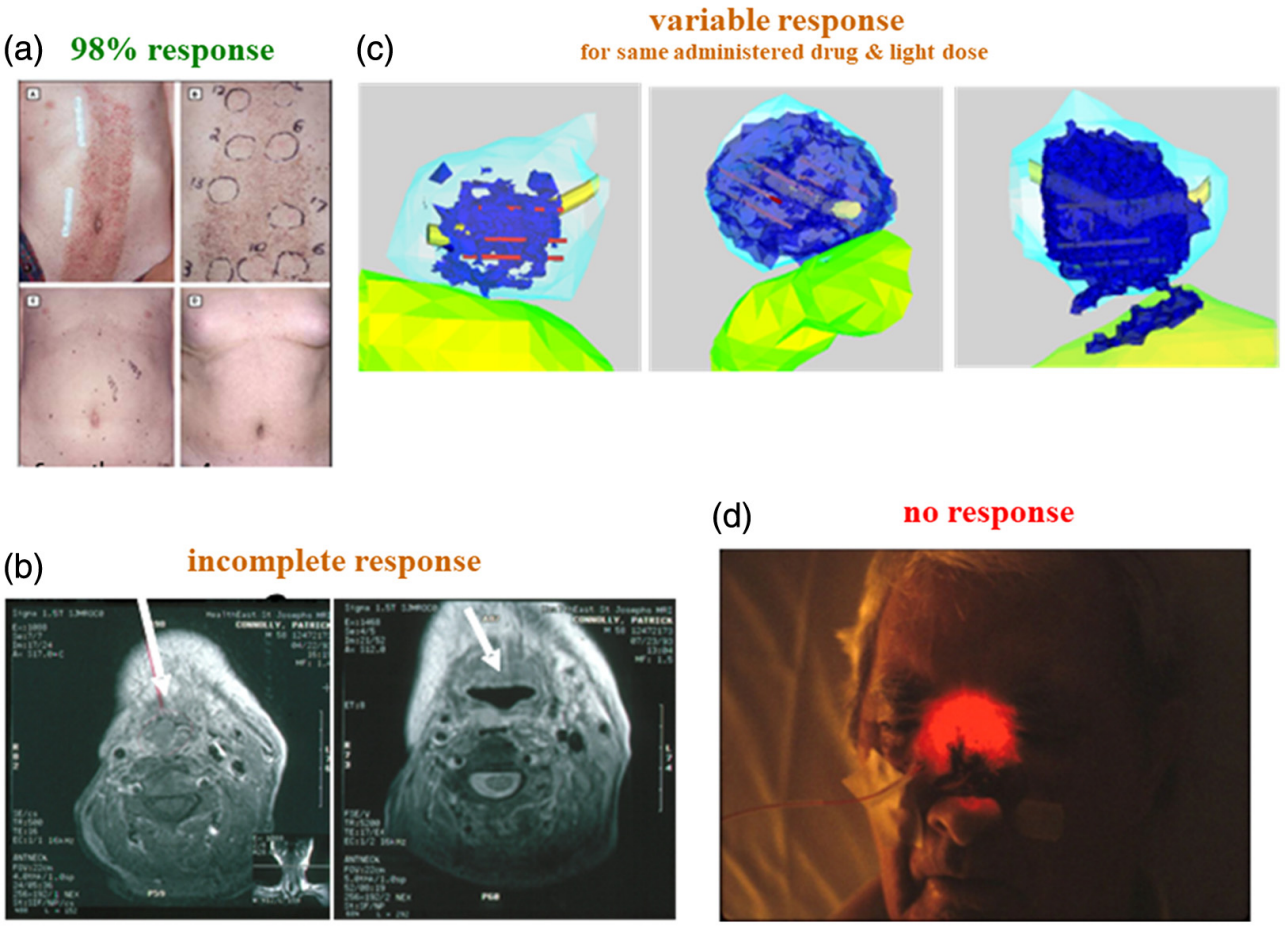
Examples of clinical cases showing large variability in response of solid tumors treated by PDT. (a) Photos of extensive basal cell carcinomas induced by prior radiation therapy: before (top left), during course of treatment showing the individual patches targeted (top right), and 6 months (bottom left) and 4 years (bottom right) after treatment with ALA-PpIX showing 98% tumor clearance.^6^ (b) CT scans of a patient with head and neck cancer before (left) and after (right) Photofrin-PDT, showing only partial tumor destruction as indicated by the white arrows (courtesy Dr Merrill Biel). (c) Reconstruction from MR images of the zones of tissue necrosis (dark blue) following PDT with a vascular-targeted type 1 photosensitizer (WST09) in recurrent prostate cancer (prostate: light blue), using multiple interstitial optical fibers for light delivery. This shows the heterogeneous PDT-induced tissue necrosis within the target volume: note also the off-target damage to the normal rectal wall in the third patient.^7^ (d) Photo during HpD-mediated PDT for recurrent nasopharyngeal cancer in which the tumor showed no treatment response (courtesy Dr. Catherine Tanser).
